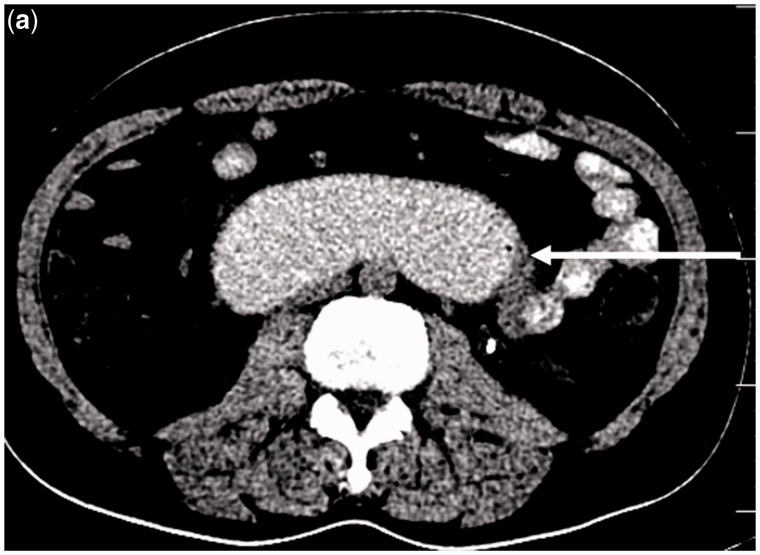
Abdomen CT scan (transverse plane): the area indicated by the arrow shows a circumferential growth seen at the region of the duodeno-jejunal flexure, also involving the medial wall of the fourth part of the duodenum. It seems to extend beyond the serosa into the adjacent mesentery.
